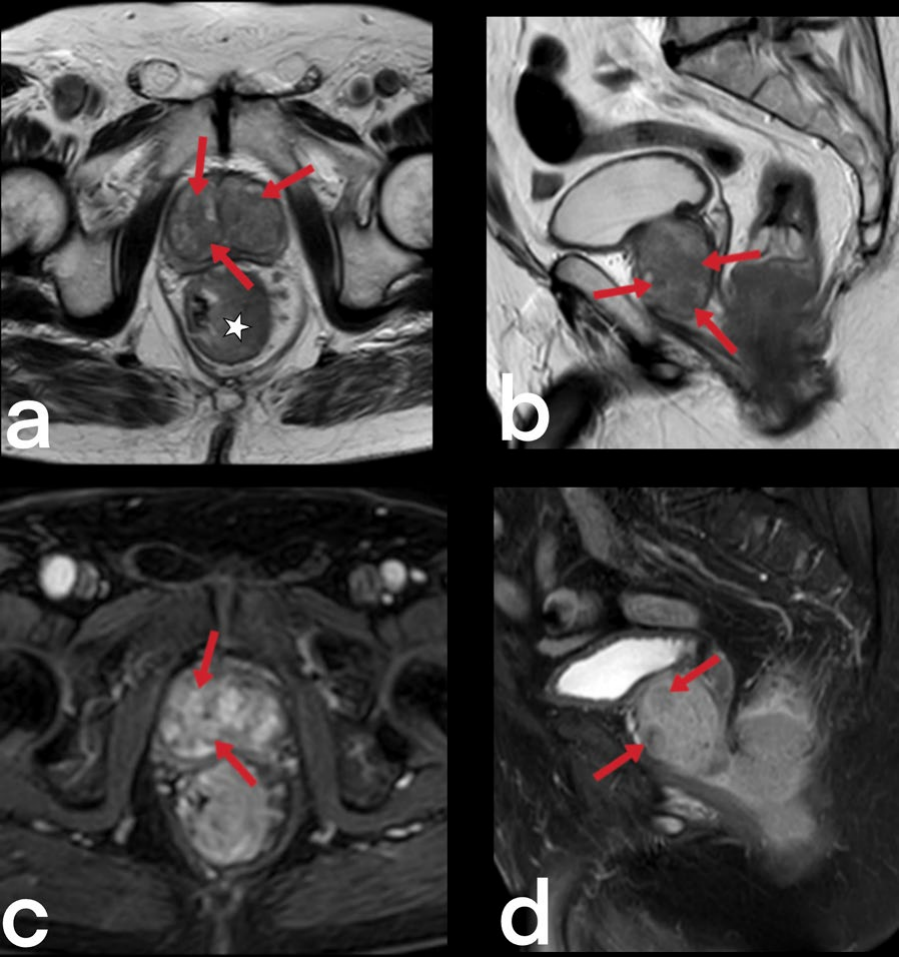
Tuberculous prostatitis (abscesses) in a 53-year-old male. T2 weighted axial (a) and sagittal (b) MR images demonstrate focal, hypointense lesions within the prostate gland (arrows). Contrast-enhancement T1 weighted axial (c) and sagittal (b) MR images show heterogeneous lesions with peripheral enhancement (arrows). A rectal tumor (star) is also seen
